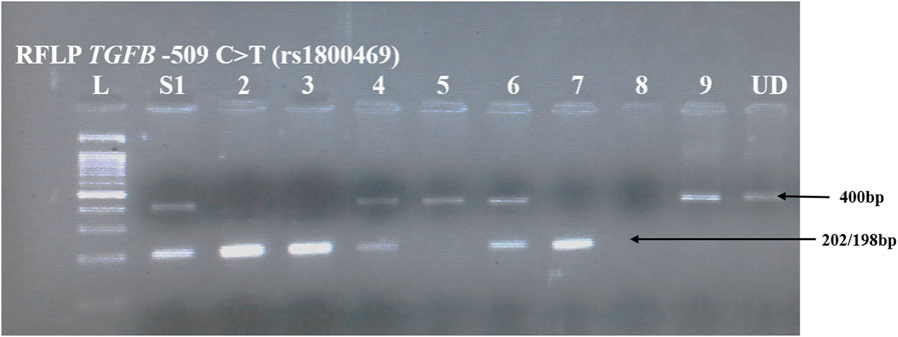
Agarose gel (2.0%) picture depicting the RFLP digested products of *TGFB* -509C > T (rs1800469) promoter polymorphism. Lane 1 consists of 100 bp molecular ladder (L); S2, S3 and S7 represents homozygous CC (198/202 bp); S1, S4 and S6 indicates heterozygous CT (400/198/202 bp) genotypes; S9 represents homozygous TT (400 bp). S8 was a failure. Last well consist of undigested PCR product (UD) of 400 bp
